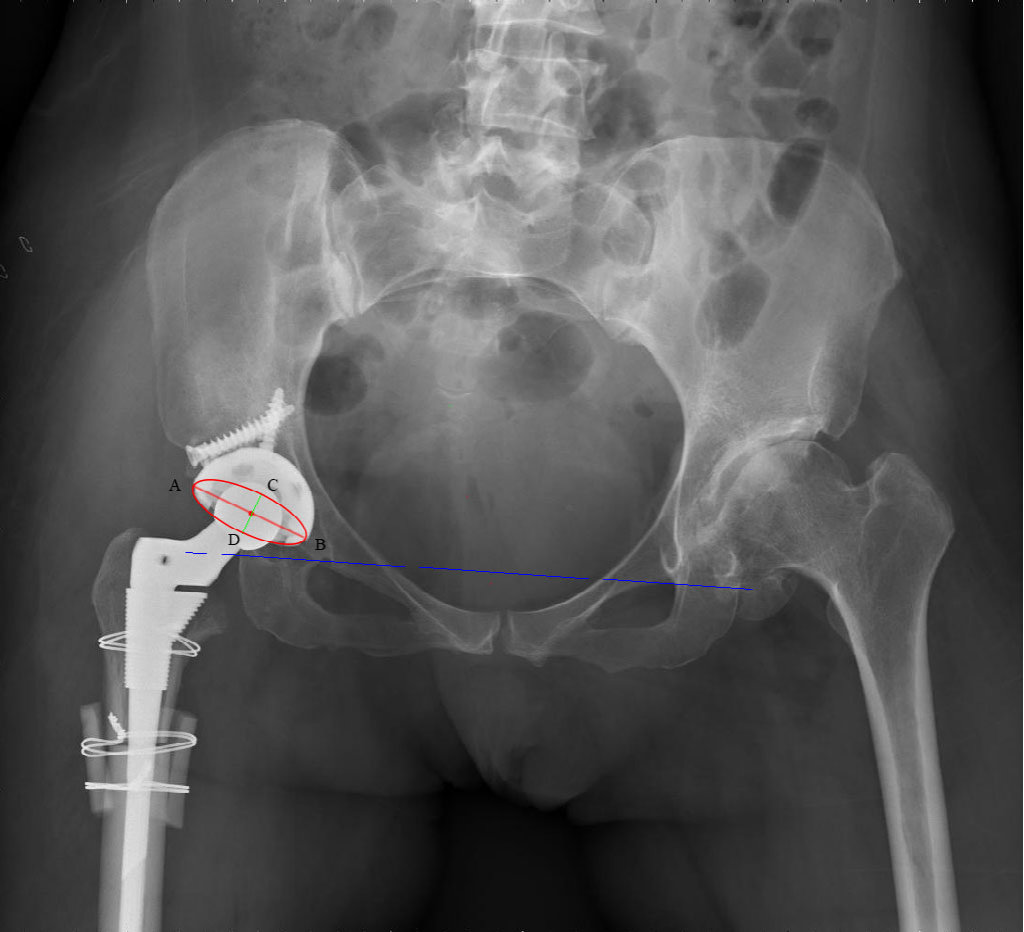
The acetabular orientation was measured on the AP pelvic radiograph. AB: long axis of the ellipse of the acetabular component. CD: short axis of the ellipse of the acetabular component. Acetabular anteversion = sin ^−1^ (CD/AB). Acetabular abduction is the angle between AB and interteardrop line (blue line).
